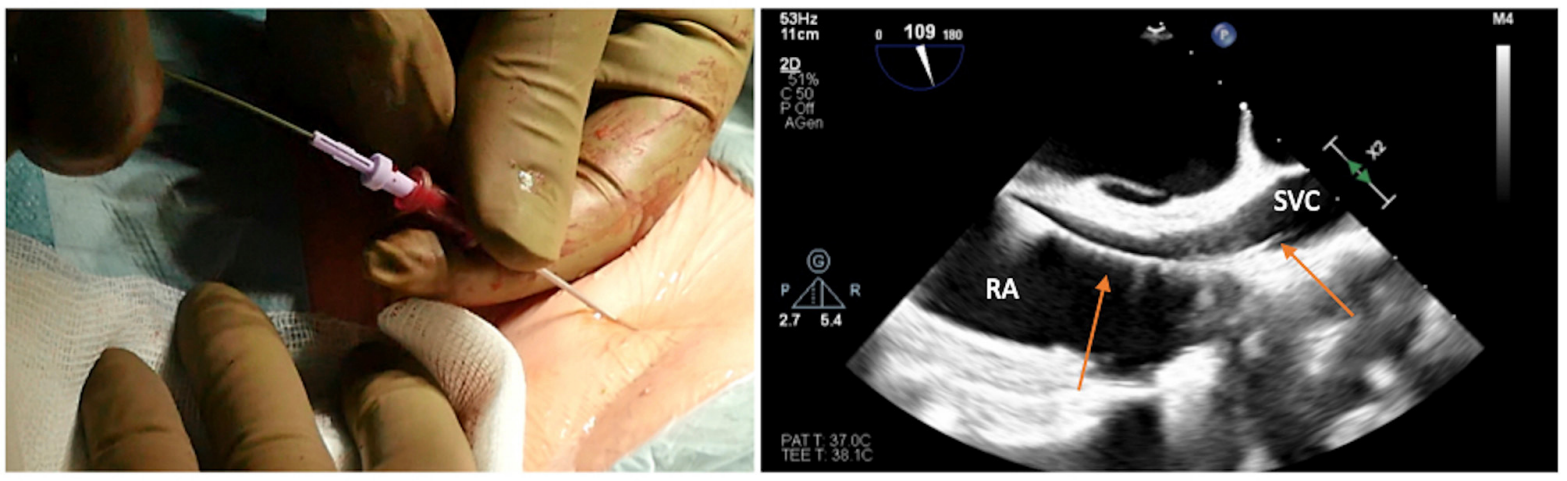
Advancing the soft J-tip guidewire through the needle up to the superior vena cavca (SVC), and visualizing the right atrial (RA)/SVC junction on transesophageal echocardiogram in bicaval projection.
